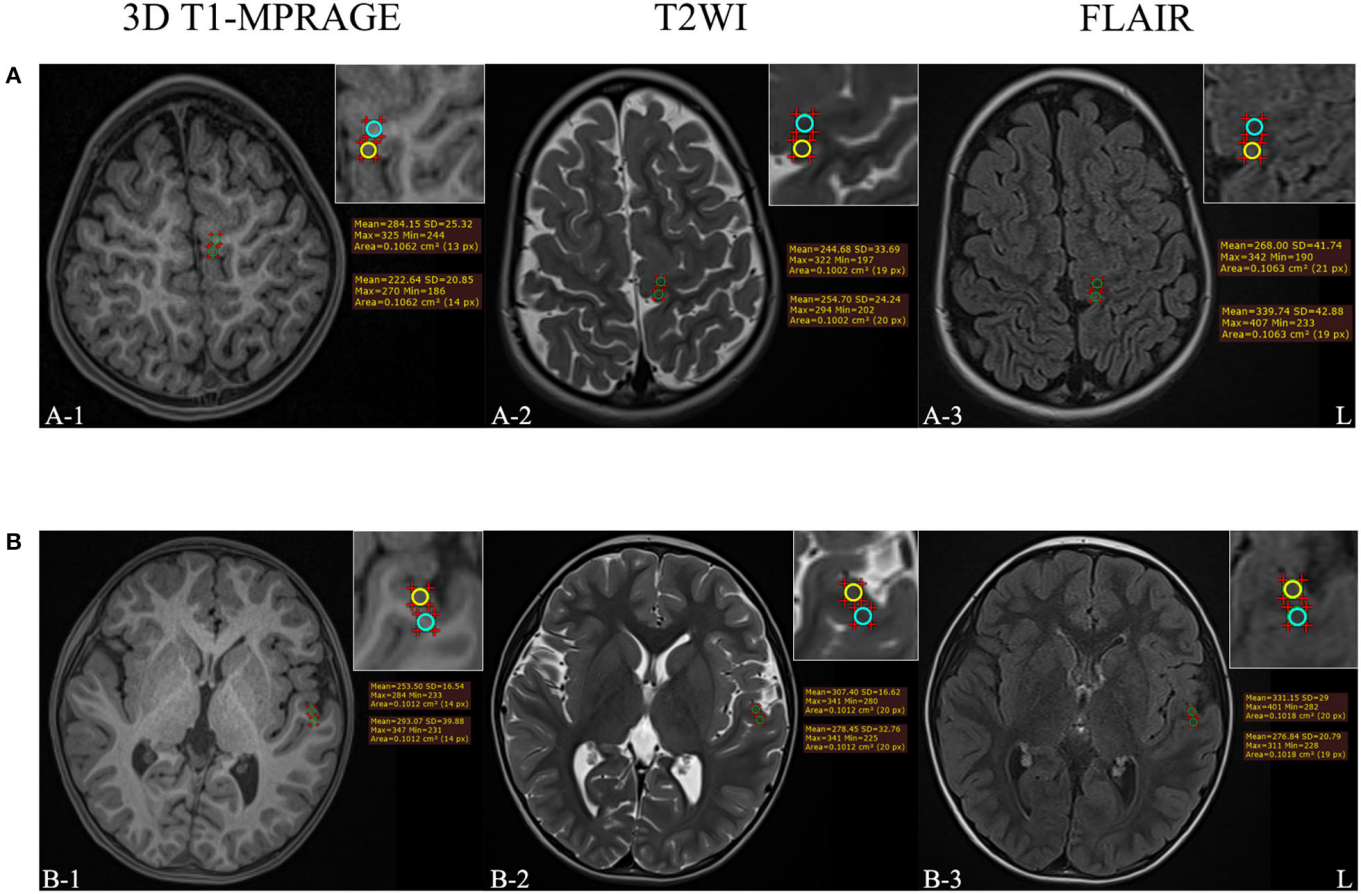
(A) The example of MRI-positive images of a 3-year-old female patient who was diagnosed with FCD IIb. (B) The example of MRI-negative images of a 4-year-old male patient who was diagnosed with FCD IIa. The upper right corner of each image was a partial enlargement. The signal intensity in the gray and white matter regions of the target lesion was measured, respectively, and the yellow circle means the ROI of abnormal gray matter while the blue circle means the ROI of abnormal white matter.
